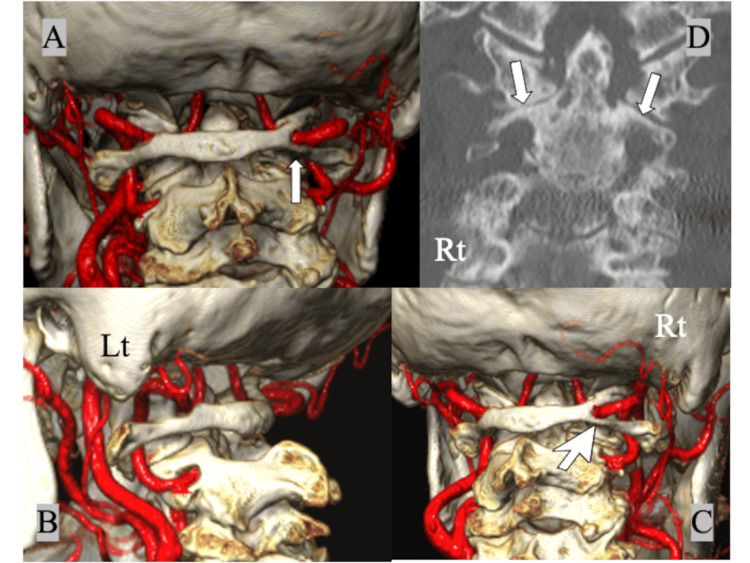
Preoperative 3D CTA showing (A) the bony bridge completely encircling the right VA on the right side (complete ponticulus posticus, white arrow), (B) a normal running course of the left VA on the VA groove, (C) a narrower posterior atlantal arch below the ponticulus posticus (white large-head arrow), and (D) bilateral high riding VAs with the thin C2 isthmus (white arrows). 3D: three dimensional; CTA: computed tomography angiography; VA: vertebral artery
